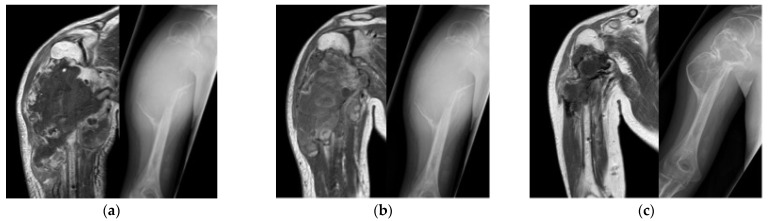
Representative case 2. The patient (patient No. 2) was referred to our hospital due to swelling of right shoulder. Based on the biopsy results, the patient was diagnosed with conventional osteosarcoma. The radiography at the initial visit (a). After induction of window therapy with CBDCA + IFO, the size of the tumor did not change (b). Thus, ADM/MTX was administered before surgery (ADM/MTX front-load). After several additional cycles of chemotherapy, the tumor responded to chemotherapy (c); however, an amputation was recommended due to the invasion of the neurovascular bundles. After surgery, the patient completed the mOS-91 regimen, and there is currently no evidence of disease. ADM, Adriamycin; CBDCA, carboplatin; IFO, ifosfamide; mOS-91, modified OS-91; MTX, methotrexate.
